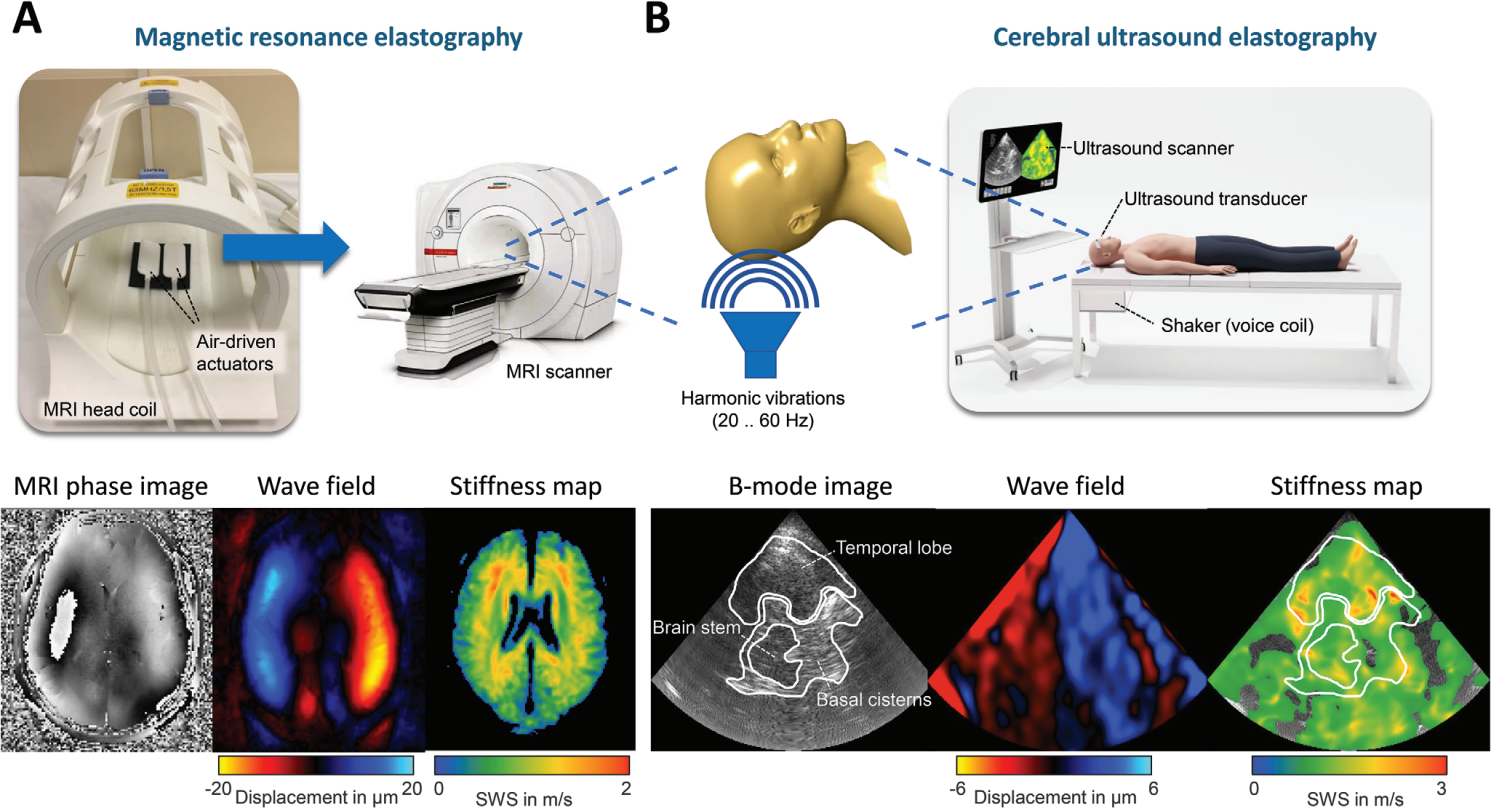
Technical setup, image acquisition, and postprocessing of brain elastography based on magnetic resonance imaging and medical ultrasound. A) Typical setup of cerebral magnetic resonance elastography (MRE). Mechanical vibration frequencies are set at the MRI console. A pressure control unit is used to transmit vibrations into the head through two actuators integrated into the radiofrequency (RF) head coil. Shear wave speed maps (SWS, in ms^−1^), a surrogate for tissue stiffness, are reconstructed using wavenumber‐based (*k*‐)MDEV inversion^[^
